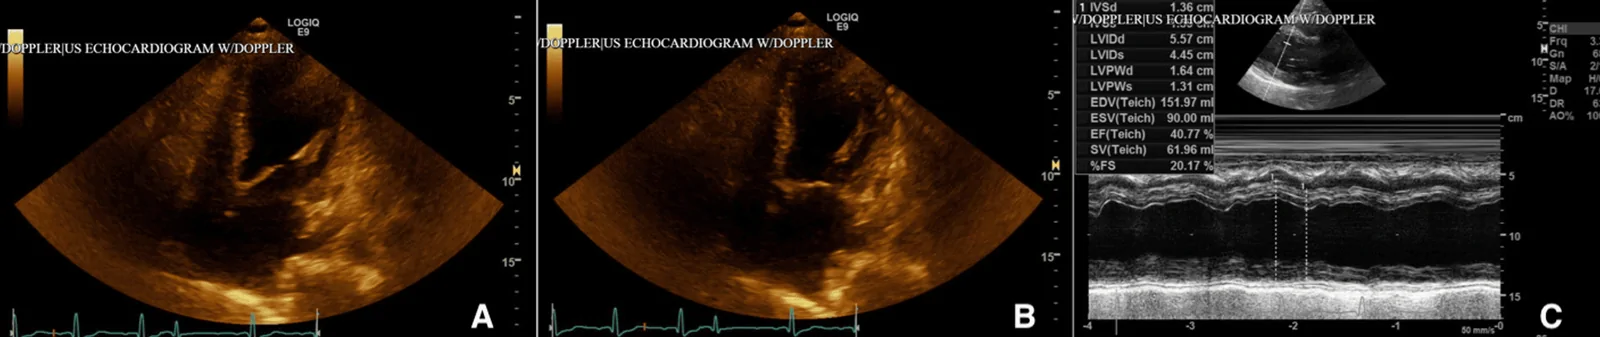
Follow-up transthoracic echocardiogram (A) End-diastolic apical four-chamber view. (B) End-systolic apical four-chamber view. (C) M-mode echocardiographic measurements demonstrating partial recovery of left ventricular systolic function, with a left ventricular internal diameter of 5.57 cm in diastole (LVIDd) and 4.45 cm in systole (LVIDs), and a calculated LVEF of 40.77%. LVIDd: left ventricular internal diameter in diastole, LVIDs: left ventricular internal diameter in systole, LVEF: left ventricular ejection fraction
